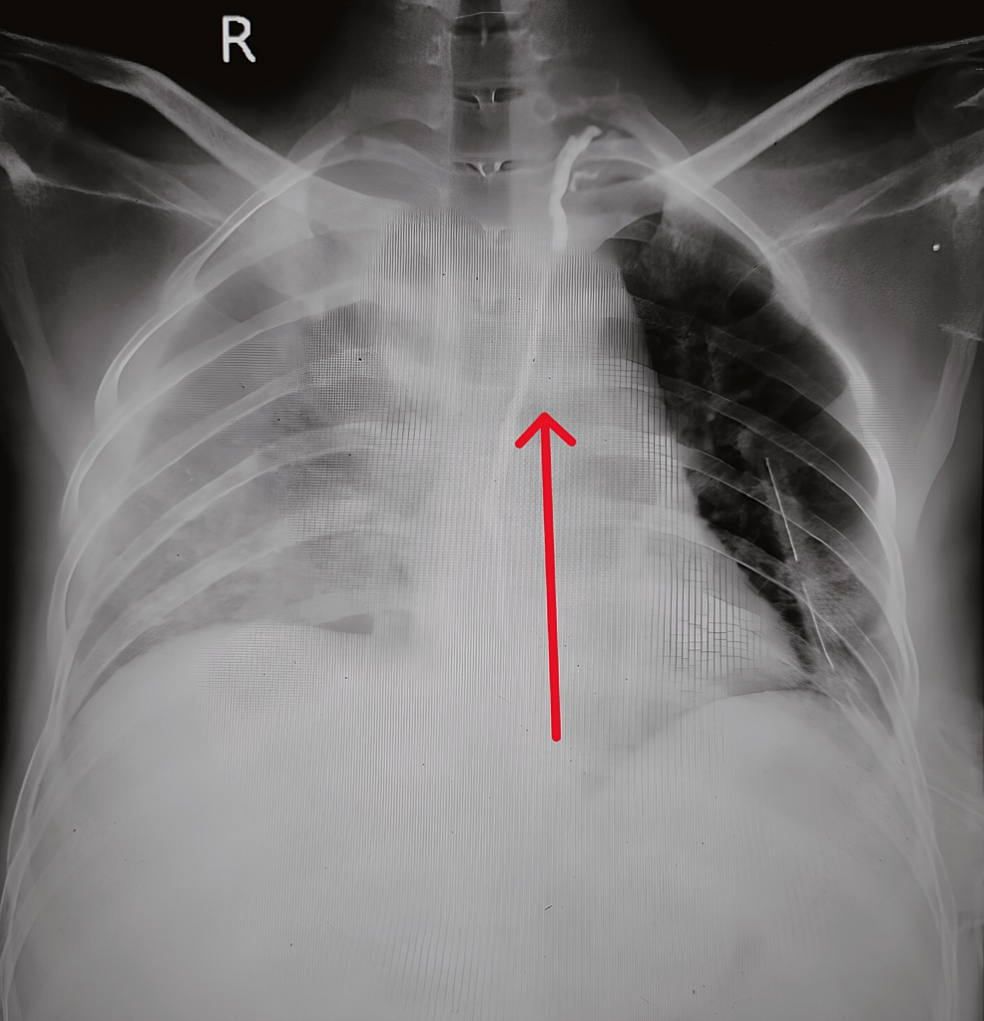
Chest x-ray anterior-posterior view post-TDE, where the embolization of the thoracic duct by cyanoacrylate glue can be seen (red arrow) TDE: thoracic duct embolization
